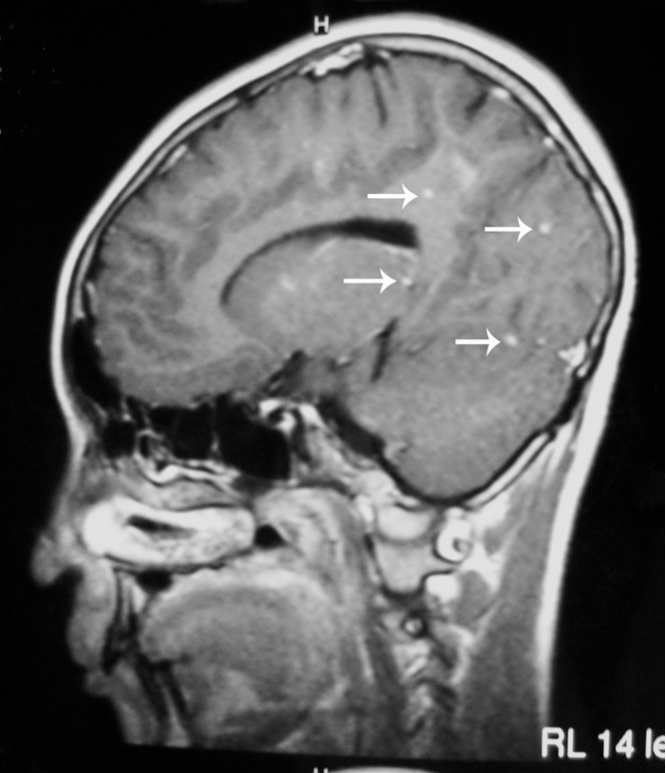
Magnetic resonance imaging of the brain of the patient, showing multiple lesions in sagittal T1-weighted images after administration of gadolinilum.
